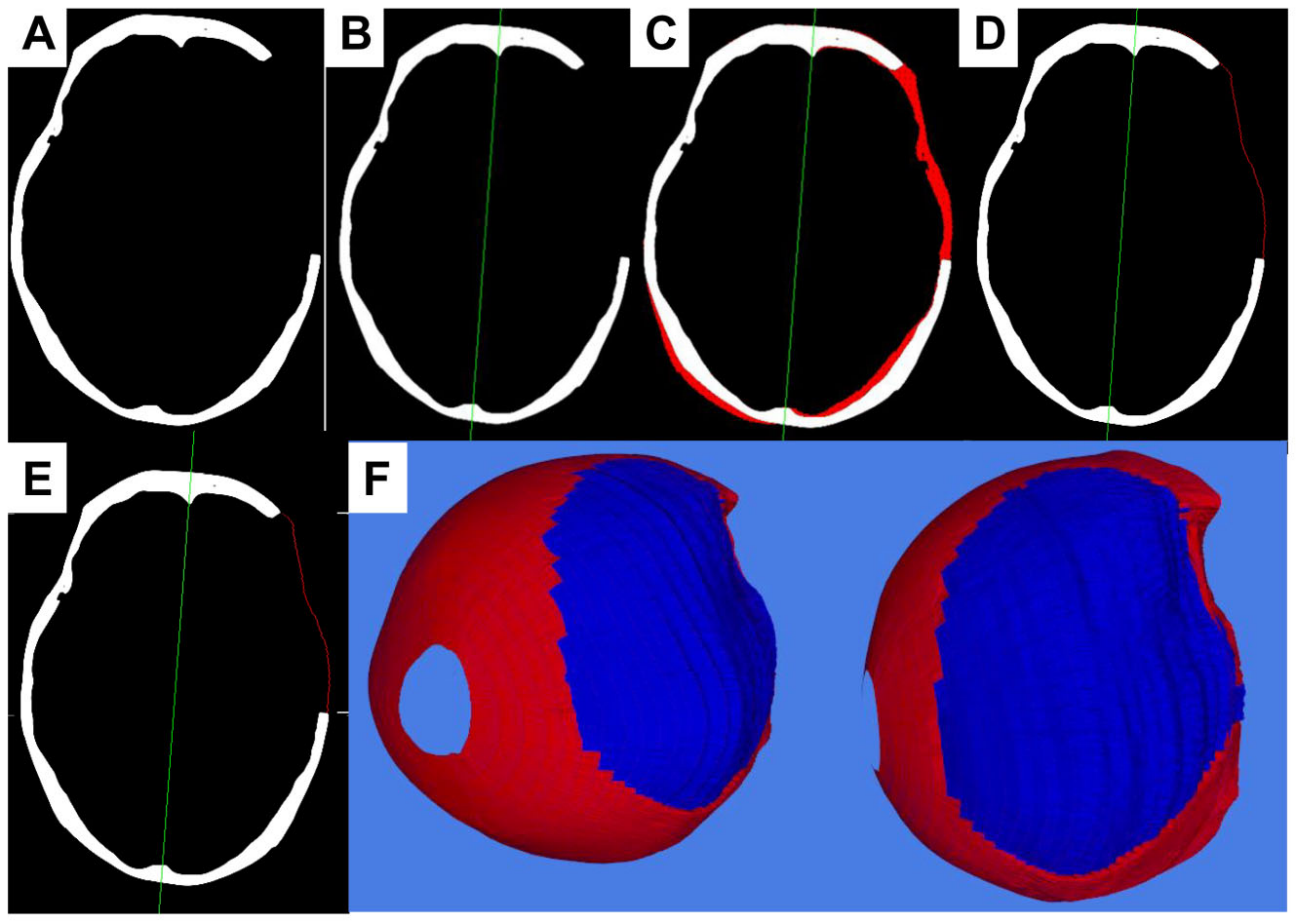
3-D CAD model for skull defect reconstruction of case 10 using OpenCV and OpenGL OBJ Viewer. (A). CT bone window image with left skull defect. (B). Axis of symmetry on CT bone window image. (C). Filled-image based on the axis of symmetry. (D). Reconstructed contour of skull defect. (E). Reconstructed contour with designated upper and lower boundaries (white line). (F). 3-D model showed by OpenGL OBJ Viewer. Reconstructed artificial flap is labeled in blue.
